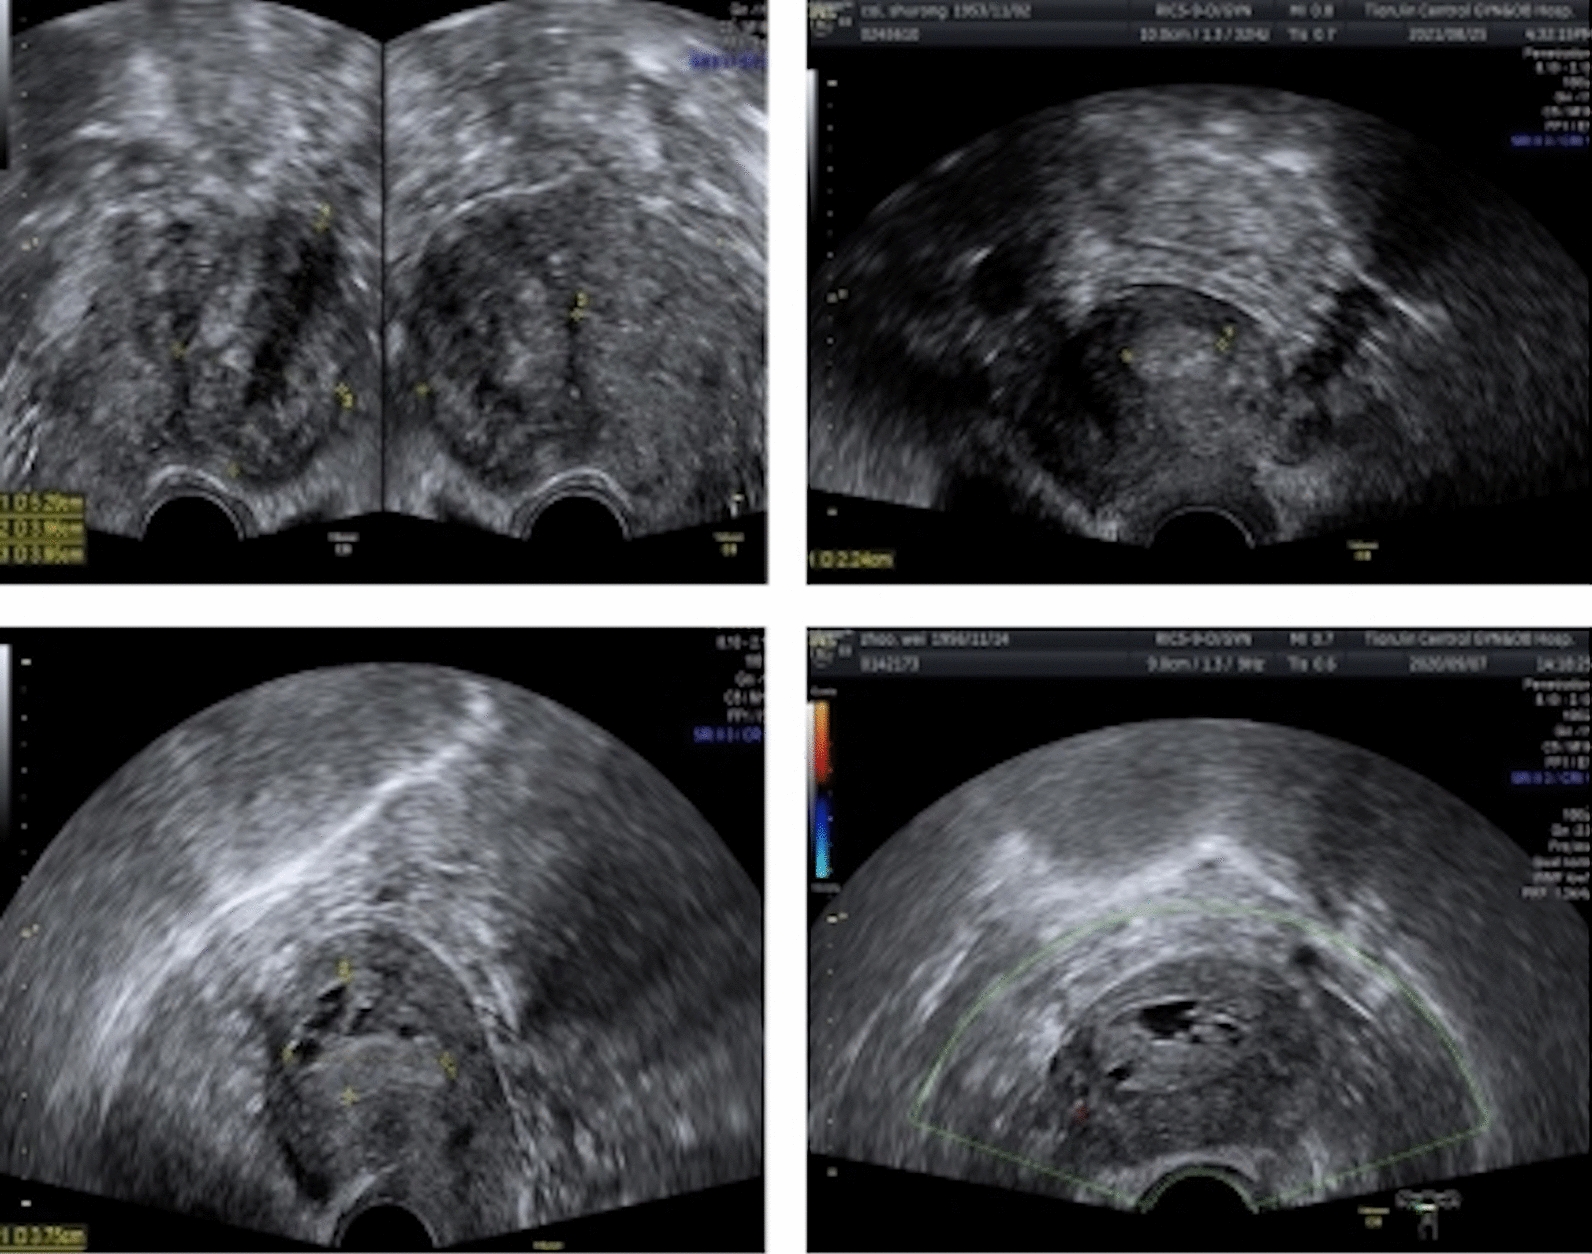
Transvaginal ultrasound image of TPA
